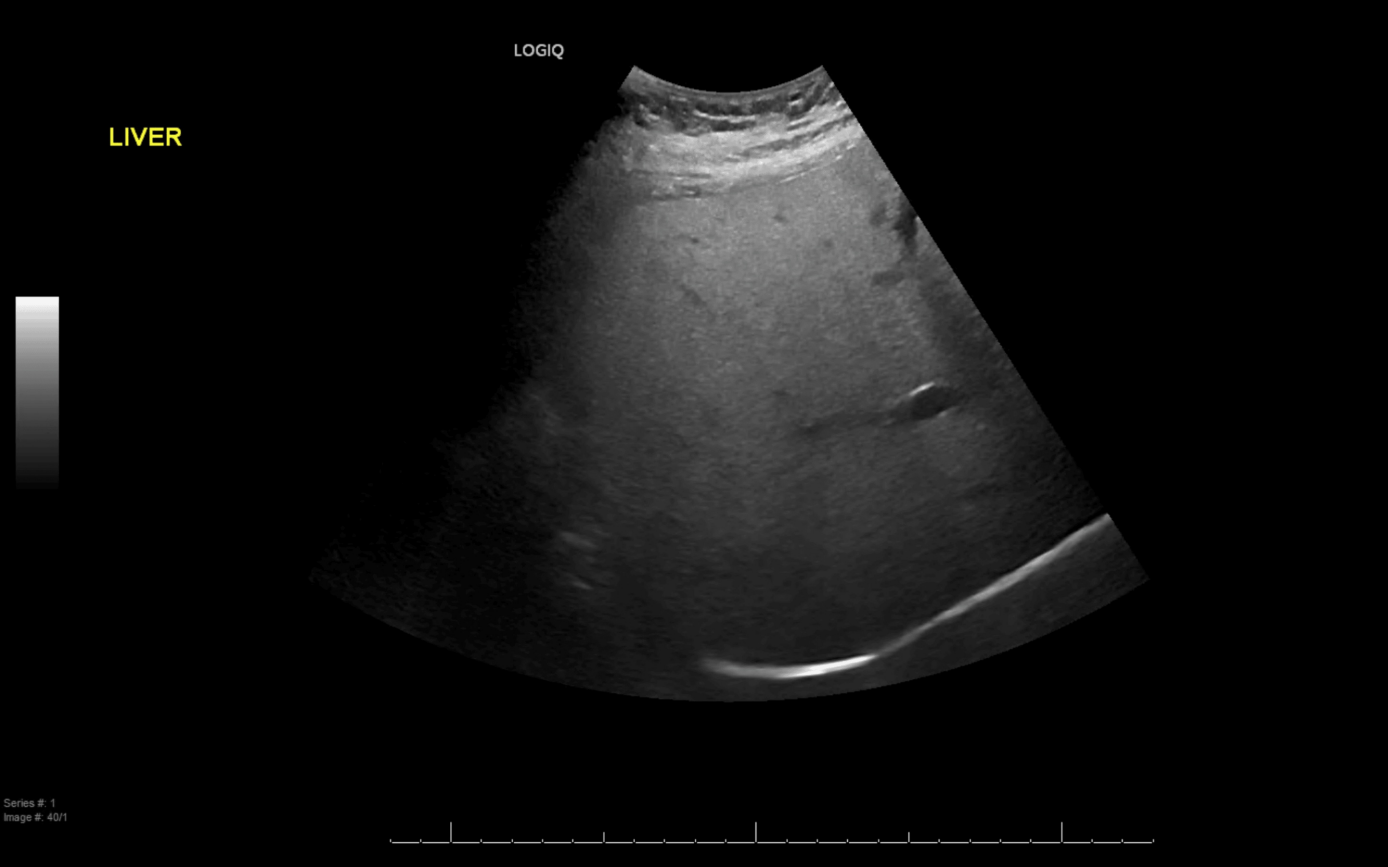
Ultrasound of the abdomen showing mild hepatomegaly with significant fatty infiltration.
